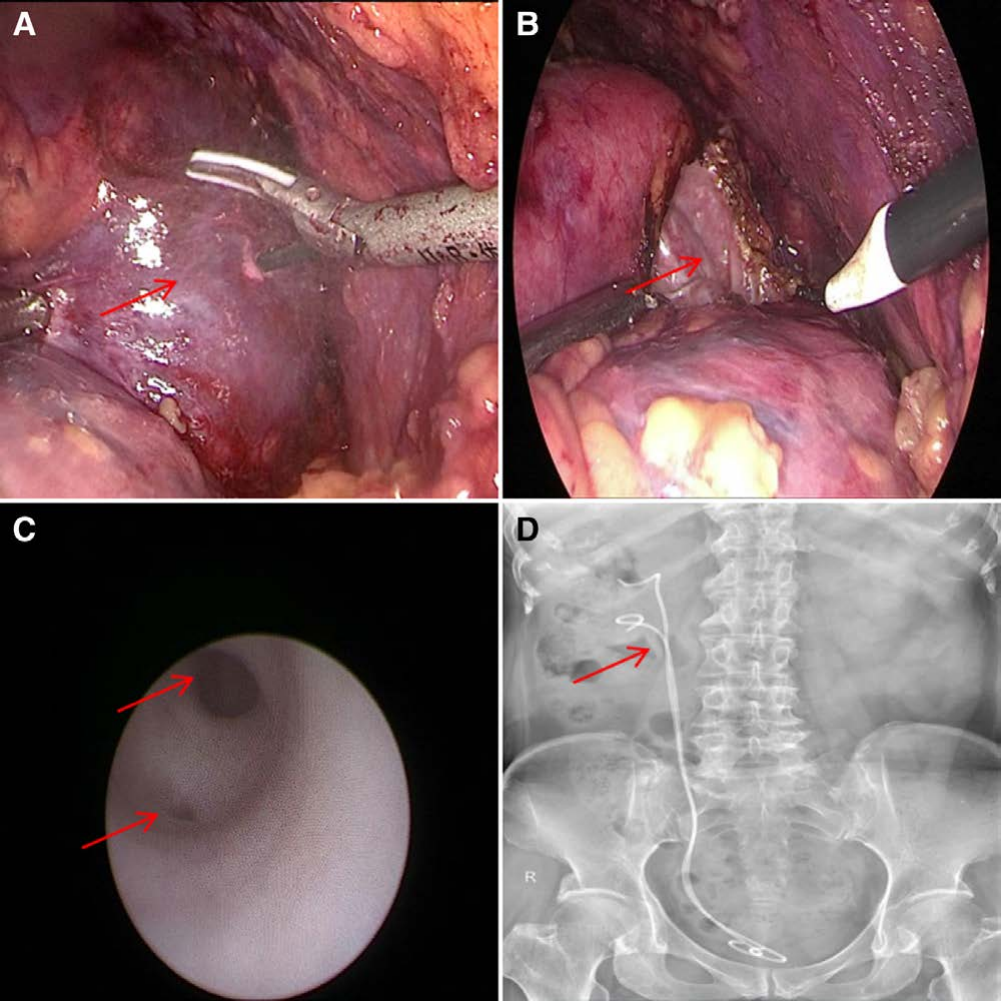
(A and B) Laparoscopic visualization of the cystic lesion in the right kidney. (C) Ureteroscopic view showing a bifurcation in the proximal segment of the right ureter. (D) Postoperative KUB radiograph following ureteral stent placement. KUB = kidney–ureter–bladder.
